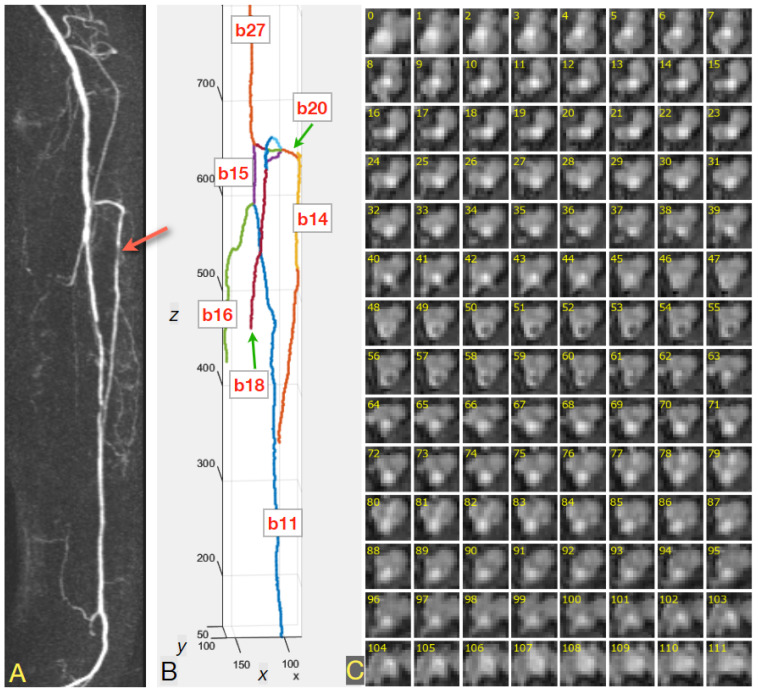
(A) Maximum intensity projection for PAVES dataset 5, showing TWIST (subtracted time-resolved acquisition) volume on the axial plane, left volunteer extremity. The arrow indicates a stenosis in the anterior tibial artery. (B) Binary skeleton of the blood vessels, after parsing. The tibial artery branch was assigned code b14. (C) Mosaic of 112 numbered cross-sections of the tibial artery, taken at 0.5-mm intervals along the centerline. An example of a coronal slice MRI of the volunteer’s right leg is shown in Figure S1.
